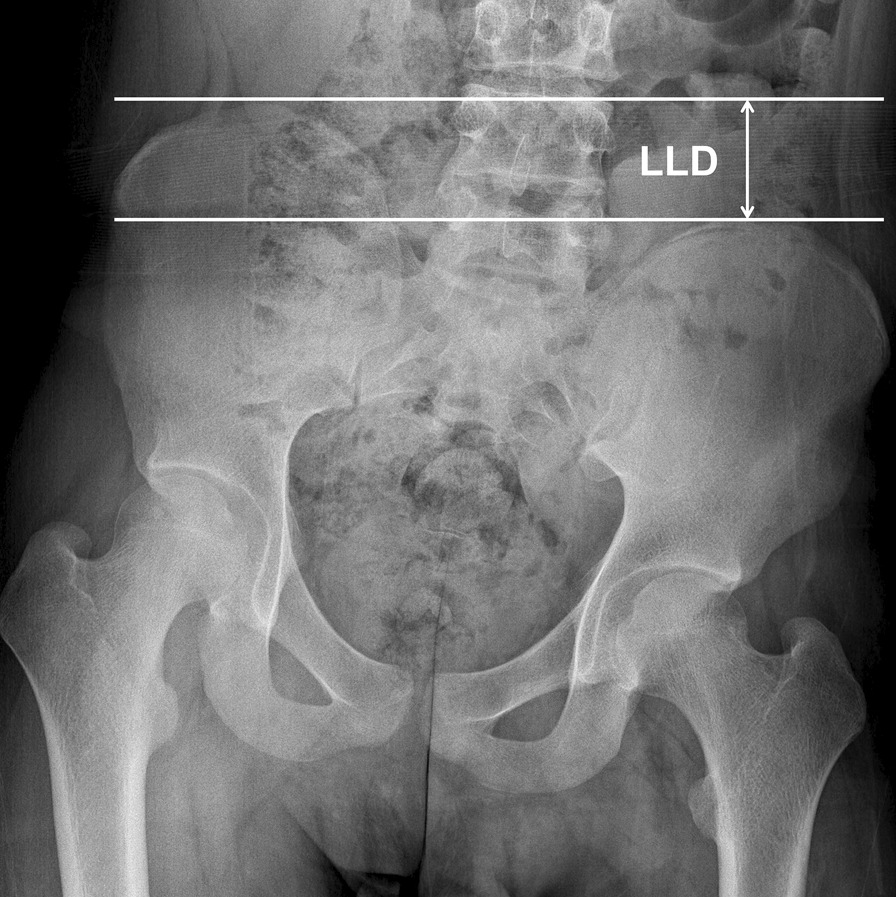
Measurement of leg length discrepancy (LLD) on a standing pelvic anteroposterior radiograph
